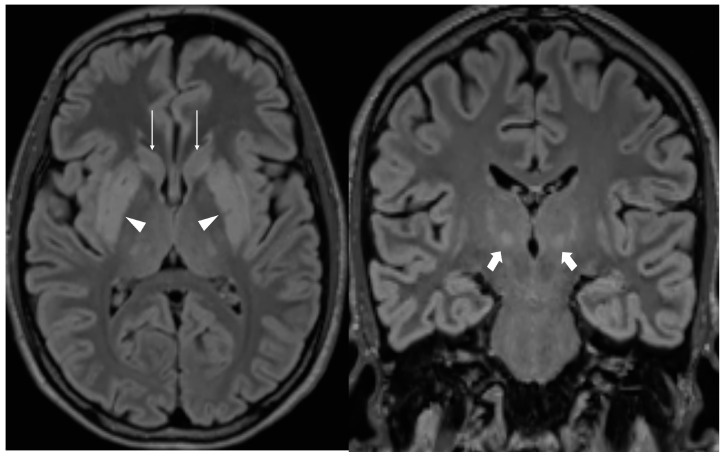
Coronal (left) and axial (right) FLAIR MRI sequences demonstrating bilateral hyperintense lesions in the putamen (arrowheads), caudate nucleus head (arrows), and thalamus (bold arrows) consistent with extrapontine osmotic demyelination syndrome.
